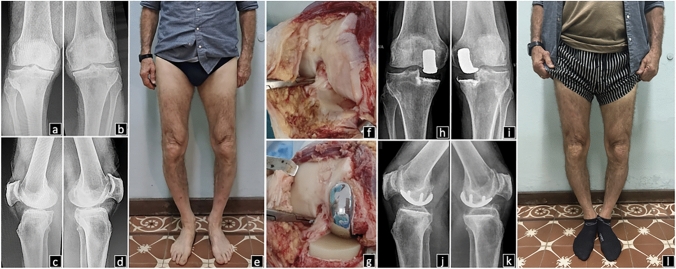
66-year-old male patient presenting with disabling bilateral knee pain that limits daily living activities. *a, b, c and d)* Preoperative anteroposterior and lateral knee radiographs. Bilateral grade 4 medial knee osteoarthritis and severe malalignment of 15º are confirmed. *e)* Varus deformity of both lower limbs. Intraoperative images of a right knee medial AP UKA. *f)* Grade 4 osteoarthritis degeneration is evident at the medial femorotibial compartment, associated with preservation of ACL and the rest of the knee compartments. *g)* Implantation of medial AP UKA as a treatment for medial femorotibial osteoarthritis. Favorable 3-year postoperative result of bilateral and simultaneous medial AP UKA. *h, i, j and k)* Postoperative anteroposterior and lateral knee radiographs showing adequate prosthetic position without signs of loosening. *l)* Restoration of the primitive limb axis after bilateral medial UKA implantation. *AP UKA* all-poly unicompartmental knee arthroplasty, *ACL* anterior cruciate ligament
